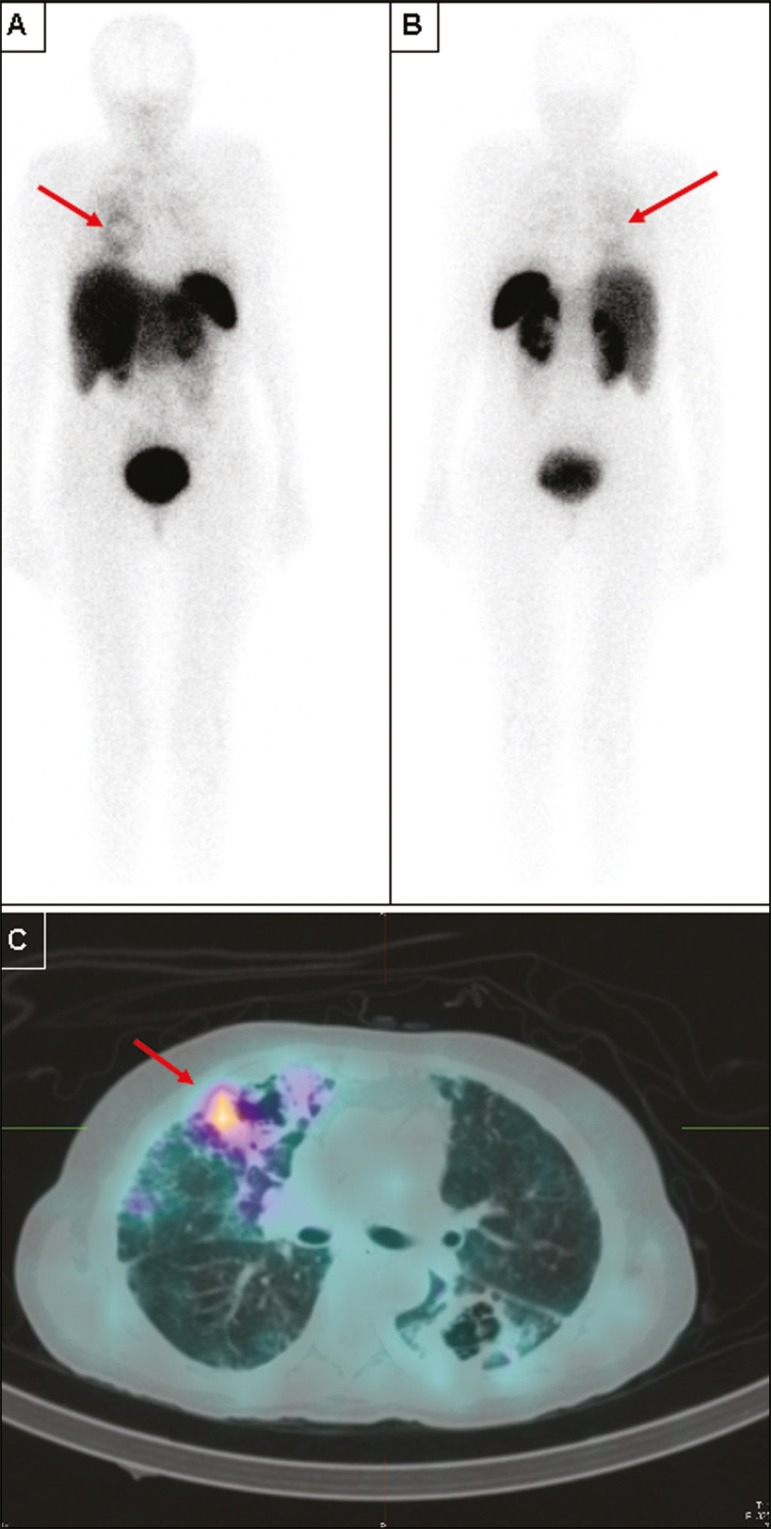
Anterior (A) and posterior (B) whole-body imaging and transaxial SPECT/CT
